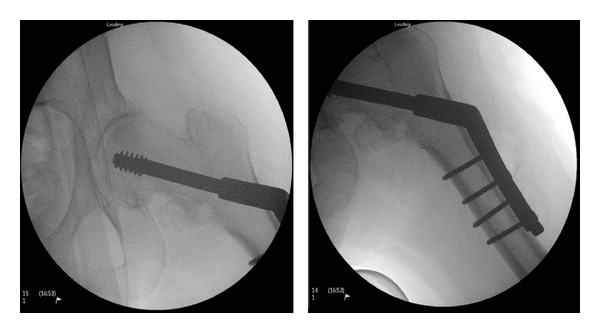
Intraoperative radiographs taken using image intensifier showing a satisfactory position of a compression hip screw.
